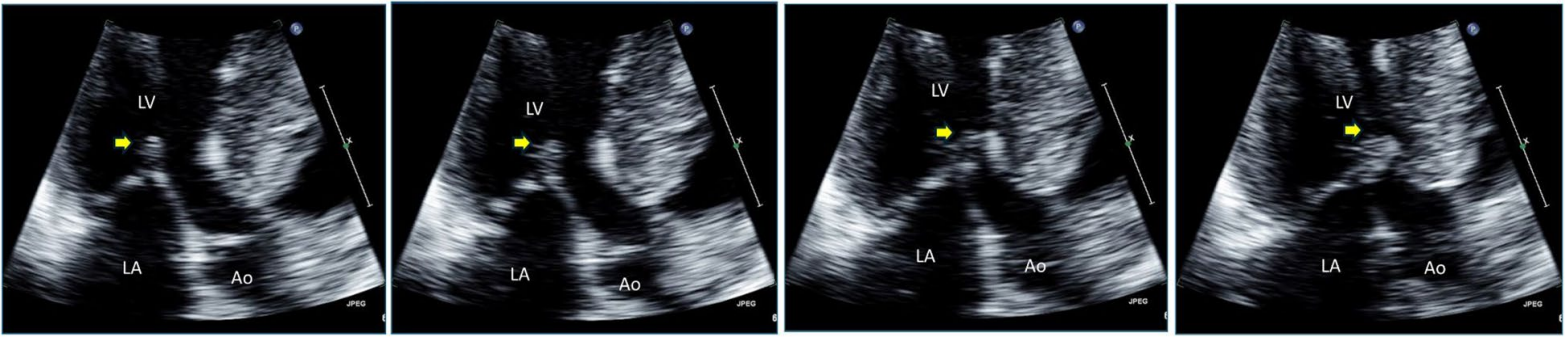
Two-dimensional echocardiographic images showing the mechanism of left ventricular outflow tract obstruction in hypertrophic cardiomyopathy (Video1). Frame-by-frame images clearly demonstrate systolic anterior motion of the mitral leaflet (arrows) resulting in septal contact and obstruction in the left ventricular outflow tract. Ao, aorta; LA, left atrium; LV, left ventricle
